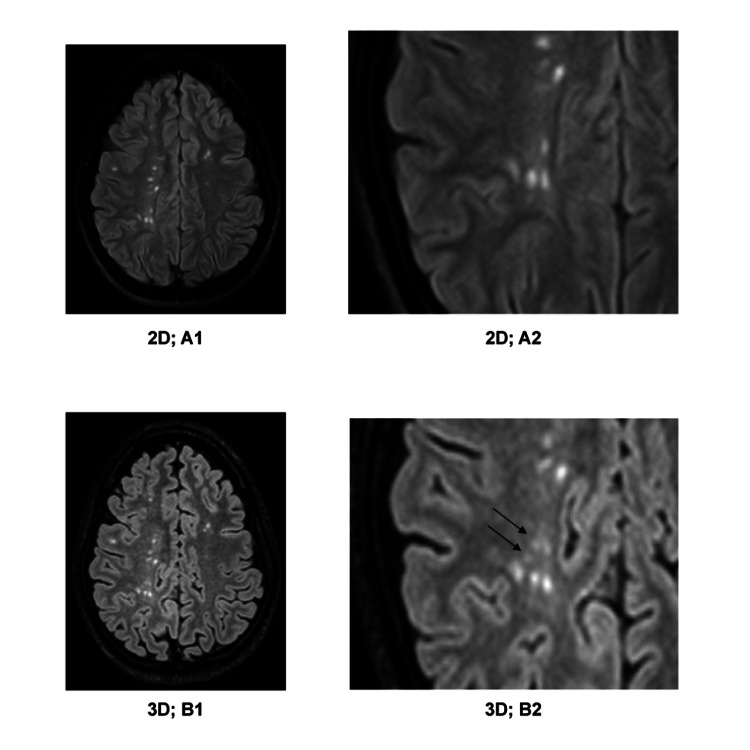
A1) 2D-FLAIR image; A2) detail enlargements of A1; B1) 3D-FLAIR image, approximately corresponding to A1; B2) detailed enlargement of B1. On the 3D-FLAIR images, white matter lesions in the right centrum semiovale (marked with arrows in B2) are visible, which cannot be detected on the 2D-FLAIR images. White matter lesions can be more clearly distinguished on the 3D-FLAIR image.
